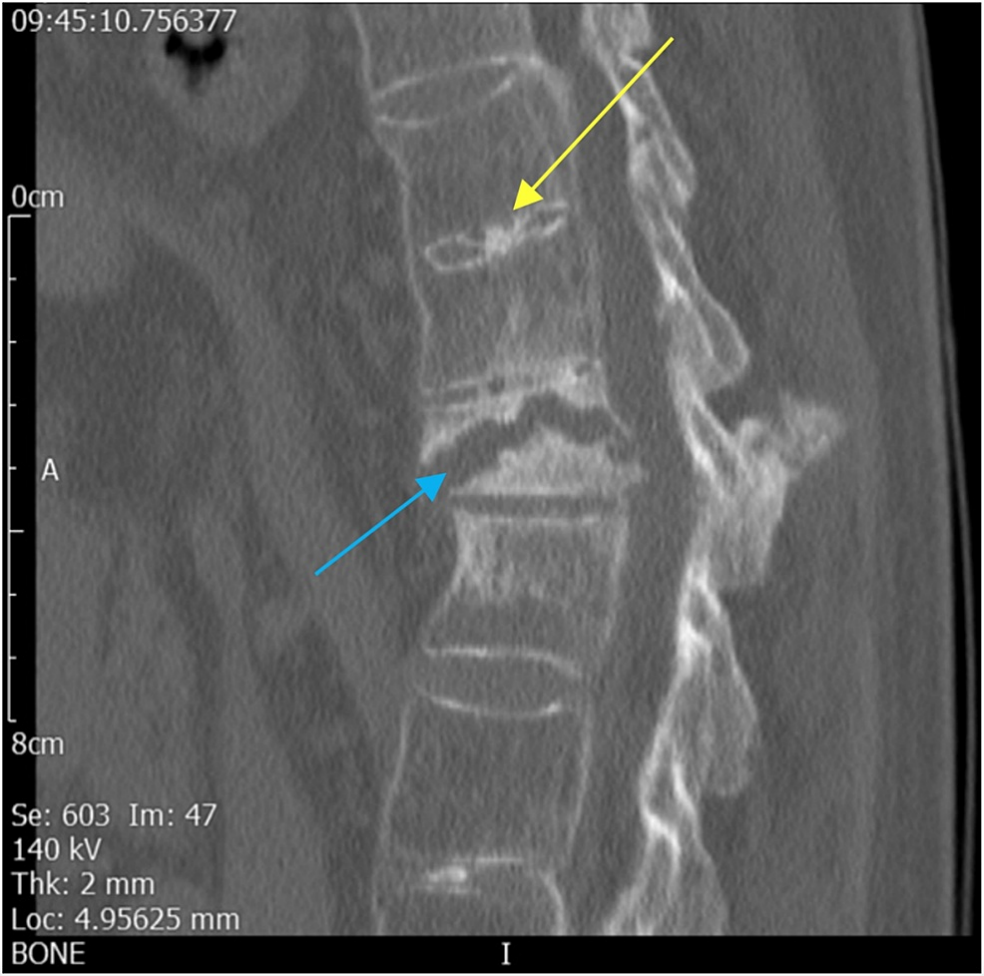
CT image demonstrating the horizontal T12 fracture to be irregular (blue arrow) with the reduction in vertebral body height, and no evidence of bony union. In keeping with the diagnosis of DISH, there is annulus fibrosus and central discal calcification (yellow arrow), associated with smooth flowing right anterolateral vertebral body osteophytosis. DISH: diffuse idiopathic skeletal hyperostosis.
